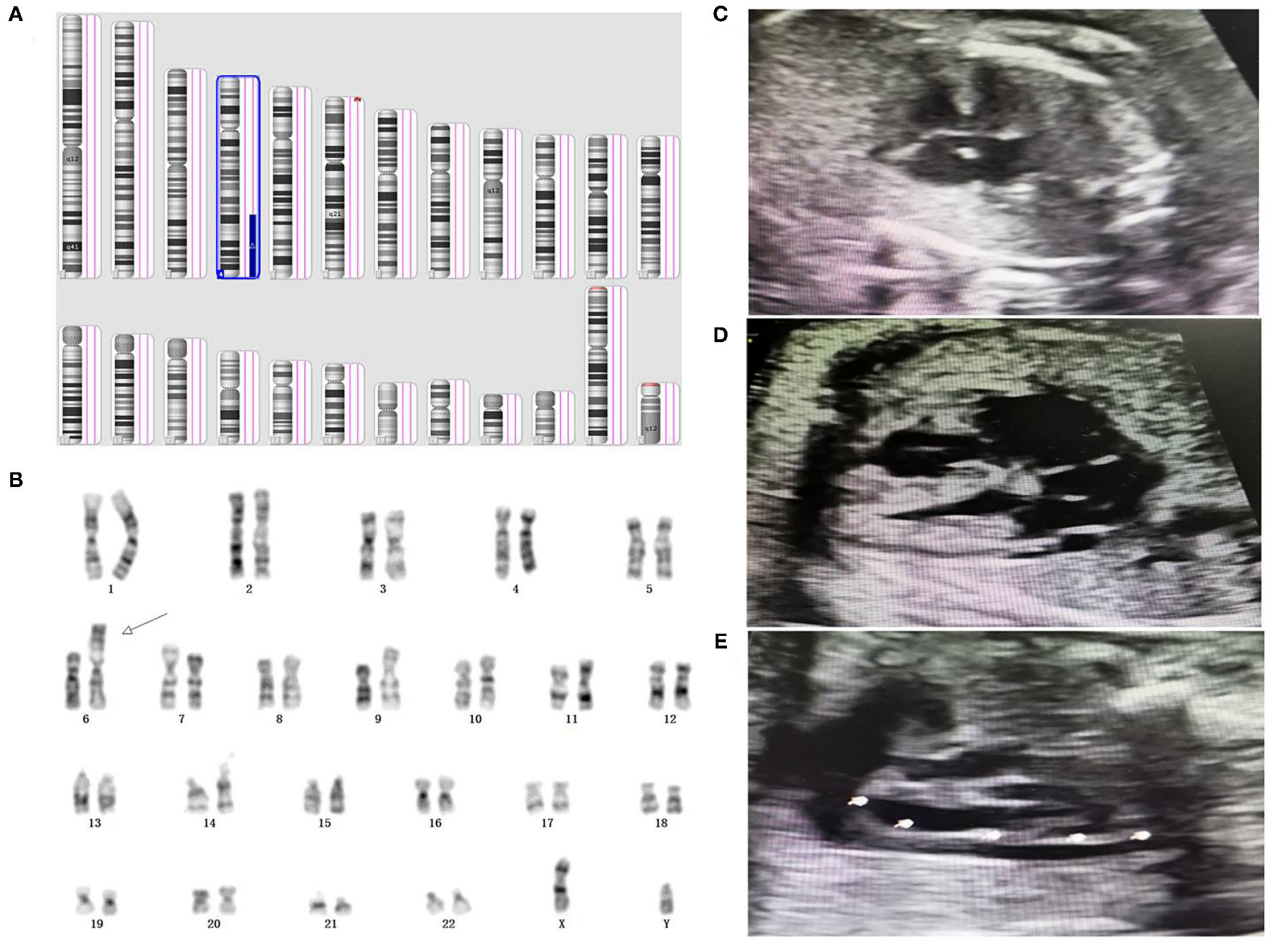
Genetic diagnosis and ultrasound phenotype of derivative chromosome 6. (A) SNP array result indicated a 4q28.3q35.2 duplication associated with 6p25.3p25.2 deletion in the fetus. (B) Karyotype analysis indicated a derivative chromosome 6 in the fetus. (C–E) prenatal ultrasound detection indicated atrioventricular septal defect, coronary sinus dilation, and persistent left superior vena cava in the fetus, respectively.
